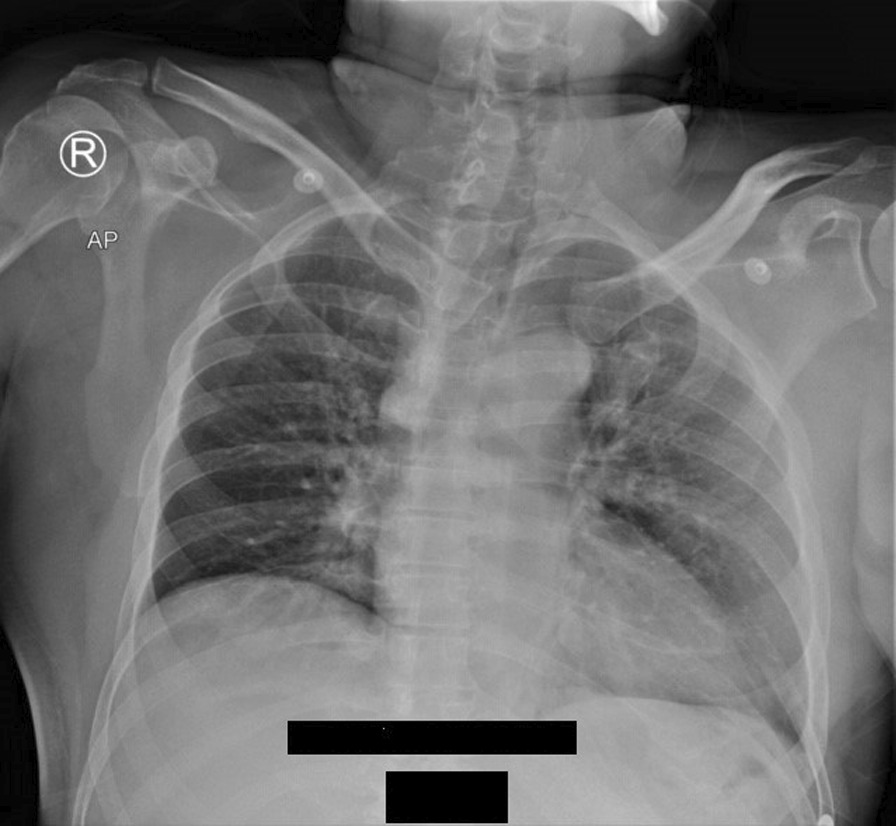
X-ray of case 1 showing bilateral airspace consolidations more prominent on the left side involving almost all zones
